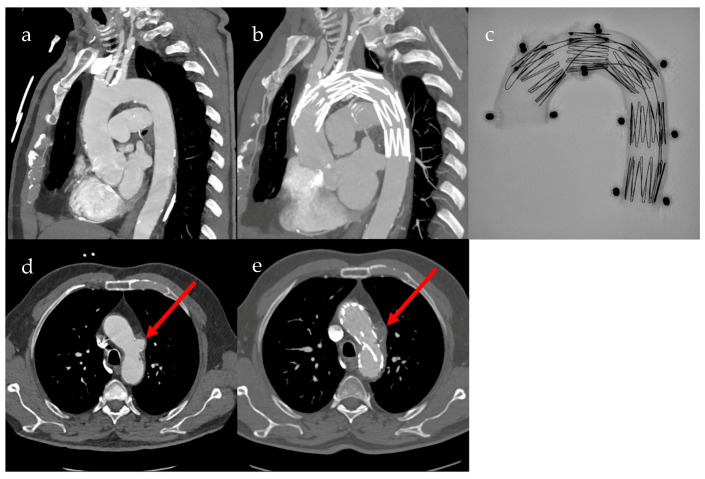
Pre- and postoperative CT scans of custom-made fenestrated thoracic endovascular aortic repair (cmfTEVAR) using the Najuta stent graft. (a) Sagittal preoperative CT image of the aortic arch; (b) sagittal postoperative CT image of the aortic arch; (c) X-ray image of the Najuta stent graft in aortic arch model; (d) transverse preoperative CT image of the aortic arch, with red arrow showing perfused penetrating aortic ulcer (PAU); (e) transverse postoperative CT image of the aortic arch, with red arrow showing non-perfused PAU.
